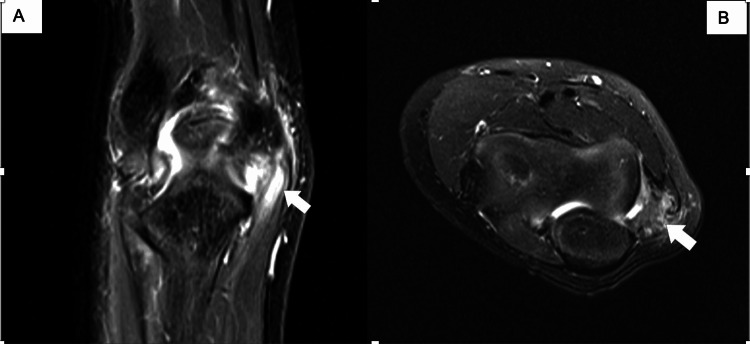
(A, B) MRI of the elbow, showing a high-grade sprain or partial tear involving the common flexor tendon (arrows), on coronal and axial views. MRI, magnetic resonance imaging
